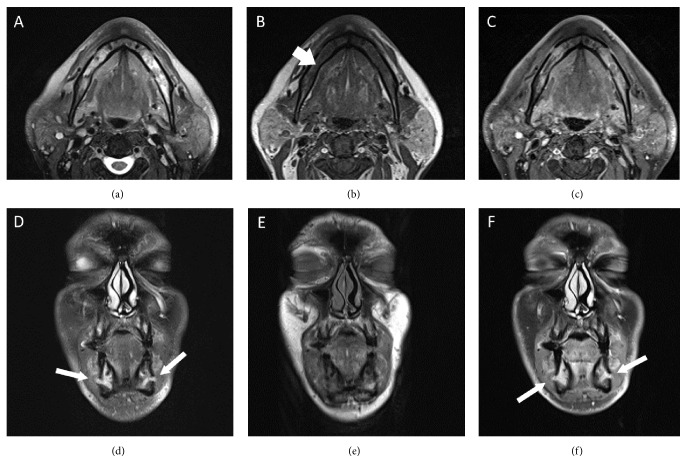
Axial MRI images at the level of the mandible demonstrating abnormal bone marrow infiltrative process replacing the normal bone signal on both T2-weighted images (a) and T1-weighted images (b) with diffuse enhancement on the contrast-enhanced axial T1-weighted images (c) (short arrows). There were no pathologic fractures and no soft tissue masses. Coronal T2-weighted images (d), unenhanced T1-weighted images (e), and contrast-enhanced T1-weighted images (f), showing abnormal thickening and enhancement of the mental nerves as they exit the mental foramen bilaterally (long arrows).
